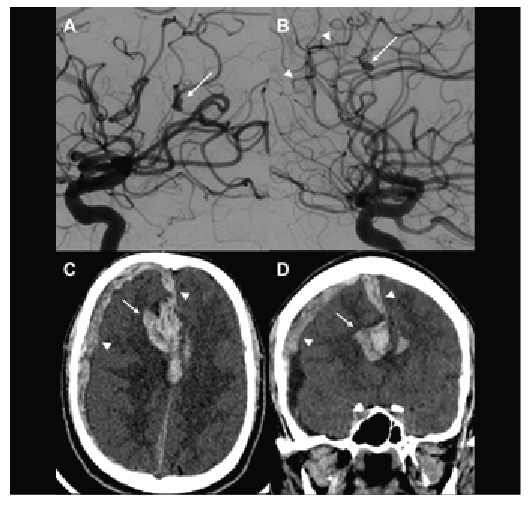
Digital subtraction angiography confirmed a fusiform aneurysm in an opercular branch of the left middle cerebral artery (arrow in A) and showed another fusiform aneurysm in the right pericallosal artery (arrow in B), consistent with mycotic aneurysms. Tiny irregularities were also observed in the frontal branches of the right anterior cerebral artery (arrowheads in B) associated with inflammatory vasculopathy. Axial and coronal head computed tomography 28 days after admission showed right frontal intraparenchymal hemorrhage (arrows) and right frontoparietal and inter-hemispheric acute subdural hematoma (white arrowheads). Uncal herniation and a downward displacement of the cerebellar tonsils (not shown) were also observed.
